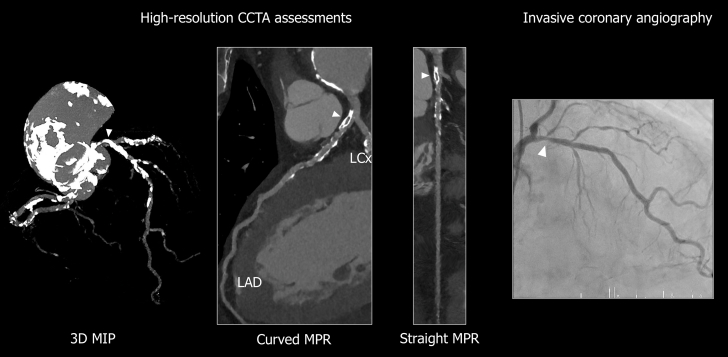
Assessment of CCTA and Invasive Angiography CCTA assessment of the lesion (arrowheads). Severe calcification extending from the distal left main trunk to the mid left anterior descending artery is clearly depicted. Invasive coronary angiography confirmed similar findings. 3D = three-dimensional; CCTA = coronary computed tomography angiography; LAD = left anterior descending artery; LCx = left circumflex artery; MIP = maximum intensity projection; MPR = multiplanar reformation.
